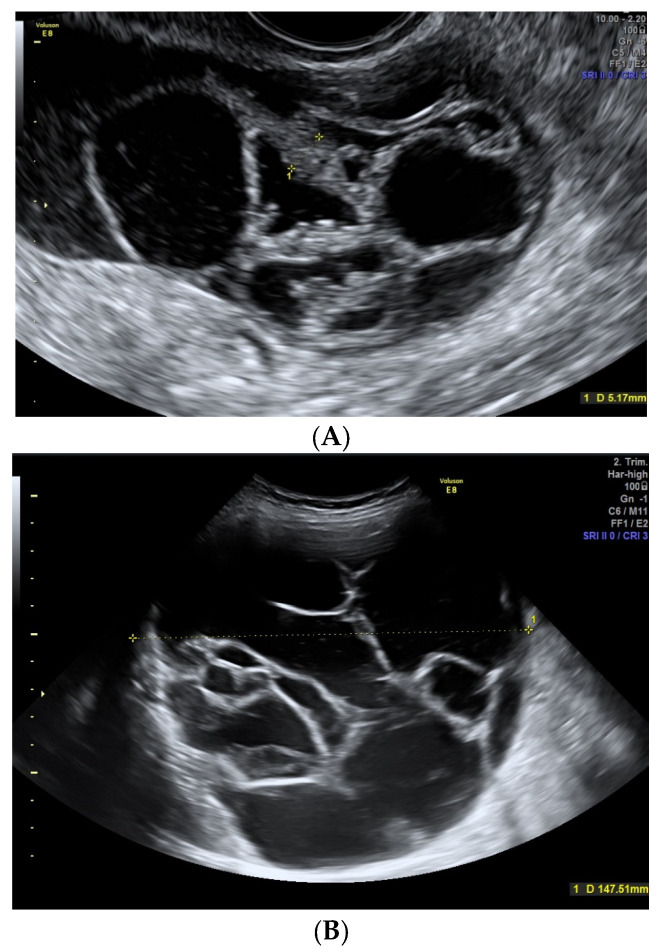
(A) Ultrasound images of a 43-year-old woman with a mucinous cystoadenoma. It shows a multilocular cyst with thick and irregular septae. (B) A 78-year-old woman with a Brenner Tumor. A big multicystic lesion is observed with thin avascular septum.
